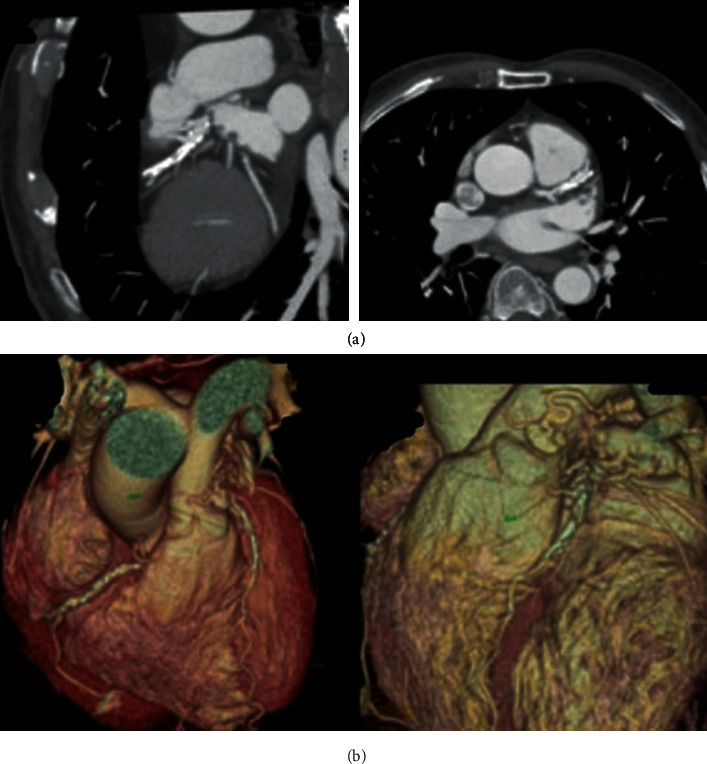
Depictions from coronary CT showing the LAD-PA fistula ((a) typical vertical sections and (b) 3D reconstruction images).
